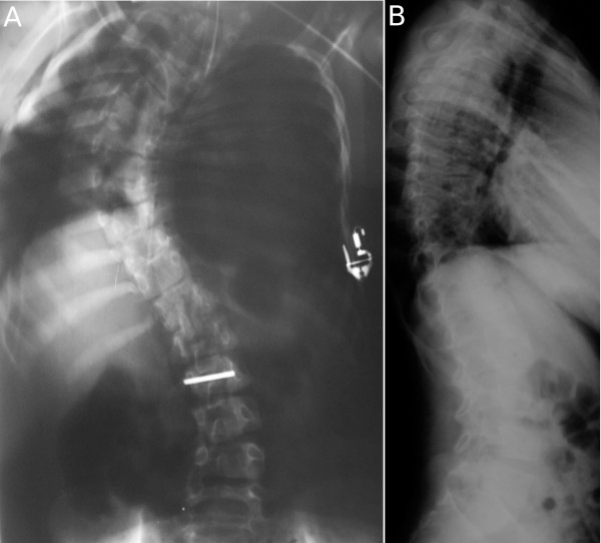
(A, B), Case 4: Spine radiographs at the age of 8, anteroposterior (A) and laterolateral (B) view.
